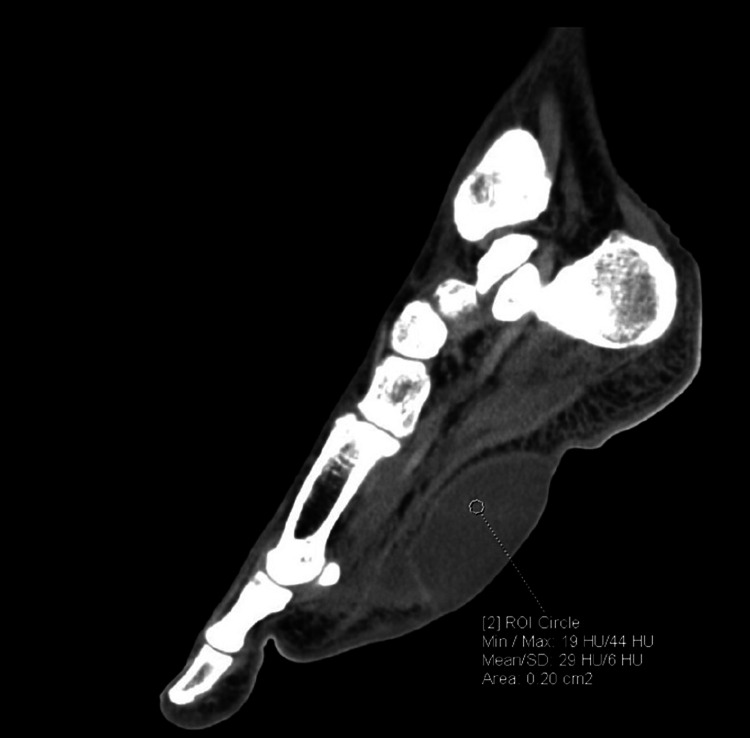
Non-contrast CT (NCCT) foot showing hypodense subcutaneous collections
